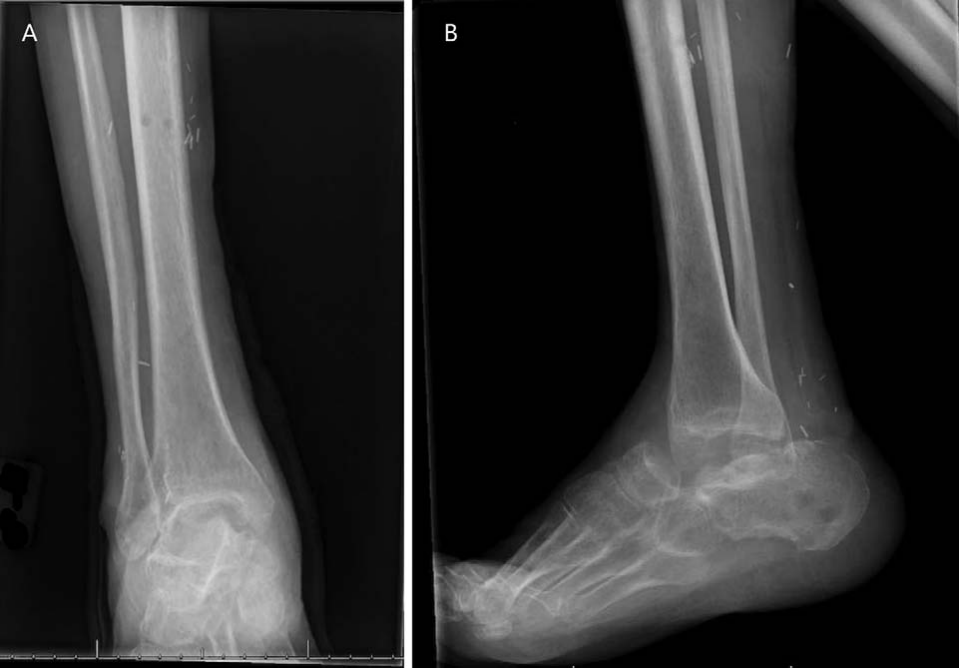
(A) Anteroposterior and (B) lateral x-rays of the patient's right ankle after antibiotic cement spacer removal.
